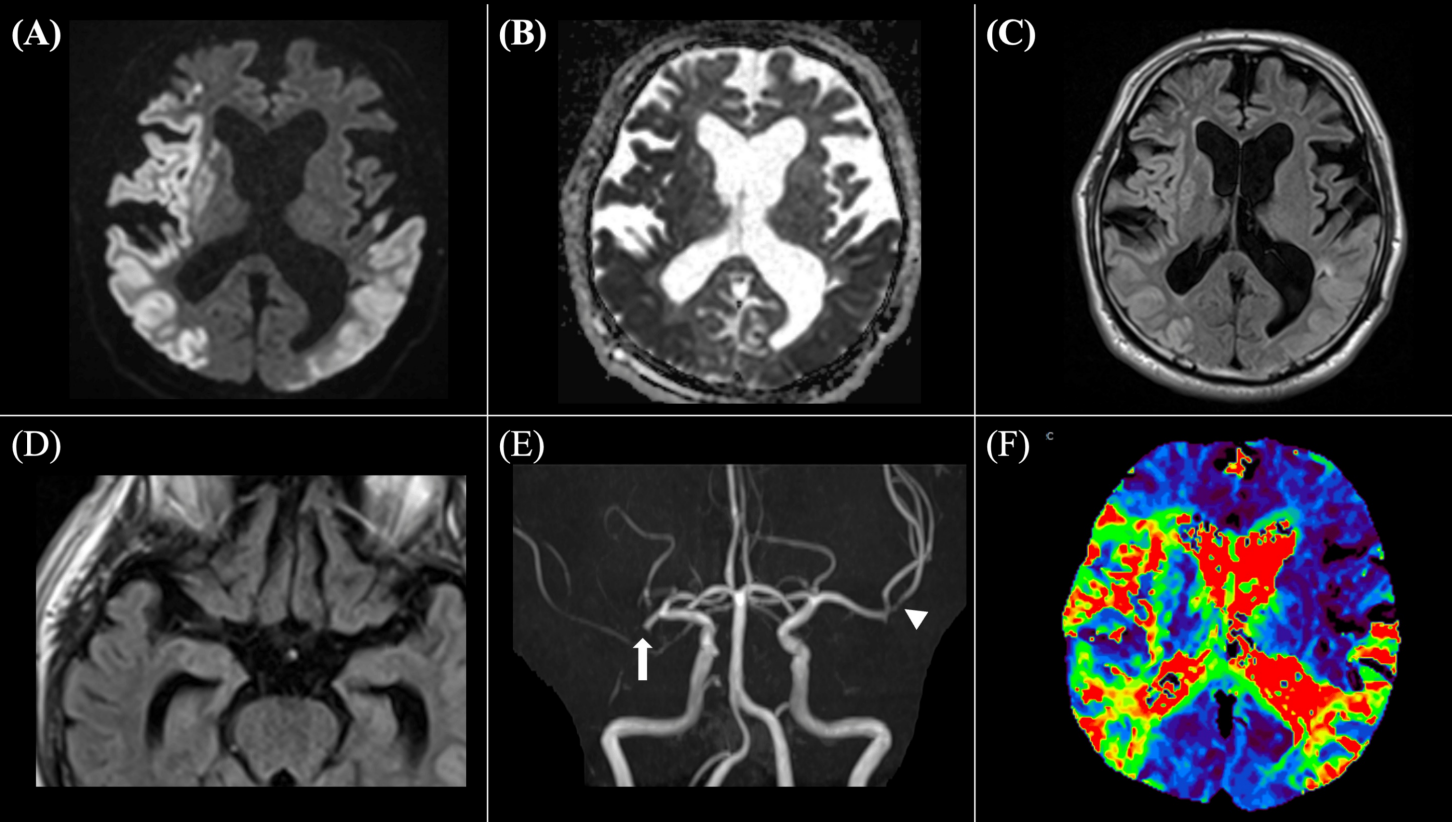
Initial magnetic resonance imaging findings on hospital admission A: diffusion-weighted imaging (DWI) shows acute-phase ischemic changes confined to the gray matter in the upper and middle trunk regions of the right middle cerebral artery (MCA) cortical branch territory and in the lower trunk region of the left MCA cortical branch; B: corresponding apparent diffusion coefficient (ADC) map demonstrates reduced ADC values consistent with infarcted area on DWI; C: fluid-attenuated inversion recovery (FLAIR) imaging reveals increased signal intensity in the infarcted regions seen on DWI, indicating vasogenic edema and evolving infarct; D: FLAIR imaging shows no intra-arterial signals in the bilateral MCAs; E: time-of-flight (TOF) magnetic resonance angiography (MRA) demonstrates a signal void at the proximal M1 segment of the right MCA (arrow) and luminal narrowing of the left MCA (arrowhead); F: perfusion-weighted imaging (PWI) using time-to-max (Tmax) maps shows perfusion deficits corresponding to the infarcted cortical regions seen on DWI, indicating impaired cerebral perfusion. Red, yellow, and green areas represent regions with prolonged transit time (>6s).
